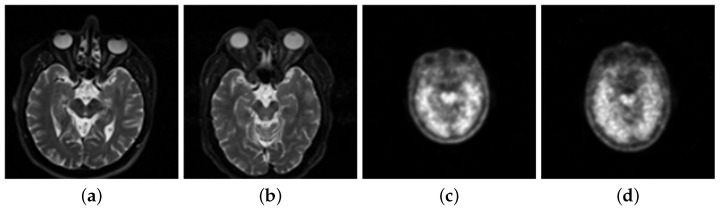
(a) Magnetic resonance image (MRI) of patients. (b) MRI of normal people. (c) Positron emission tomography (PET) image of patients. (d) PET image of normal people.
